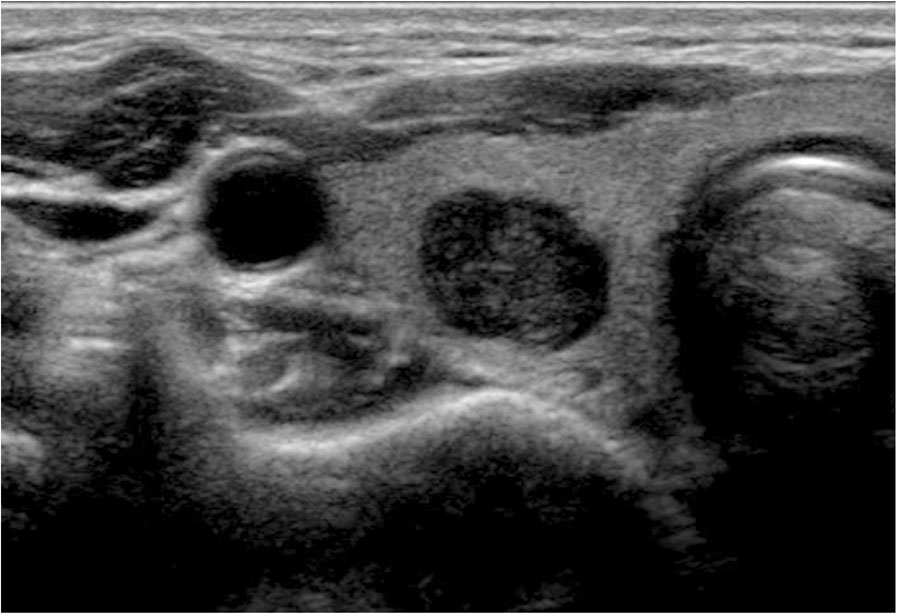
The sonographic features of ovoid to round shape, marked hypoechogenicity, solid composition, and smooth margin were shown on a transverse ultrasound image from a 40-year-old female with MTMC
